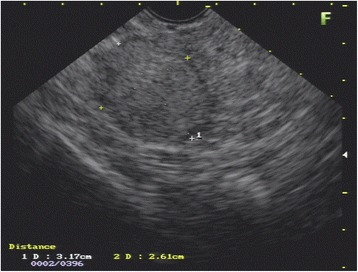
Endoscopic ultrasound scan showing a solid, heteroechoic with predominantly hypoechoic areas, well-defined lesion with regular contours (measuring 3.1 × 2.6 cm), localized between the head and neck of the pancreas
